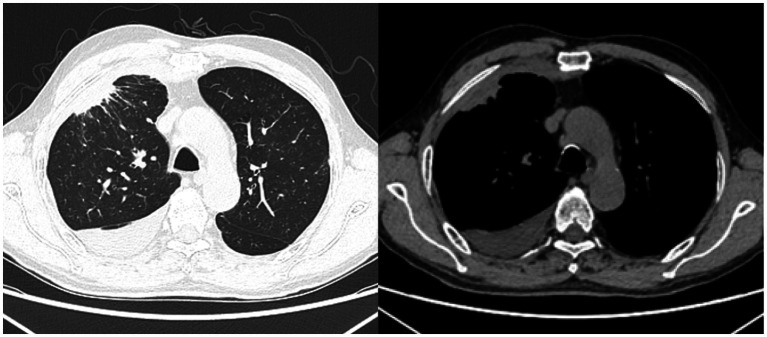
Chest CT on admission: there are patchy lesions observed in the upper lobe of the right lung, in conjunction with a right-sided pleural effusion accompanied by pleural thickening.
